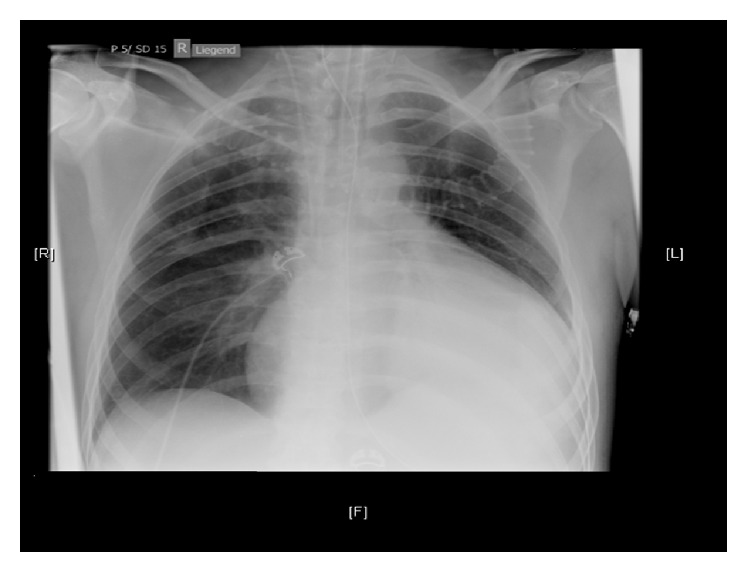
Chest radiograph (anteroposterior view) shows no pulmonary infiltrates or effusions, no pneumothorax, and no cardiac congestion but an enlarged heart with a water-bottle configuration.
